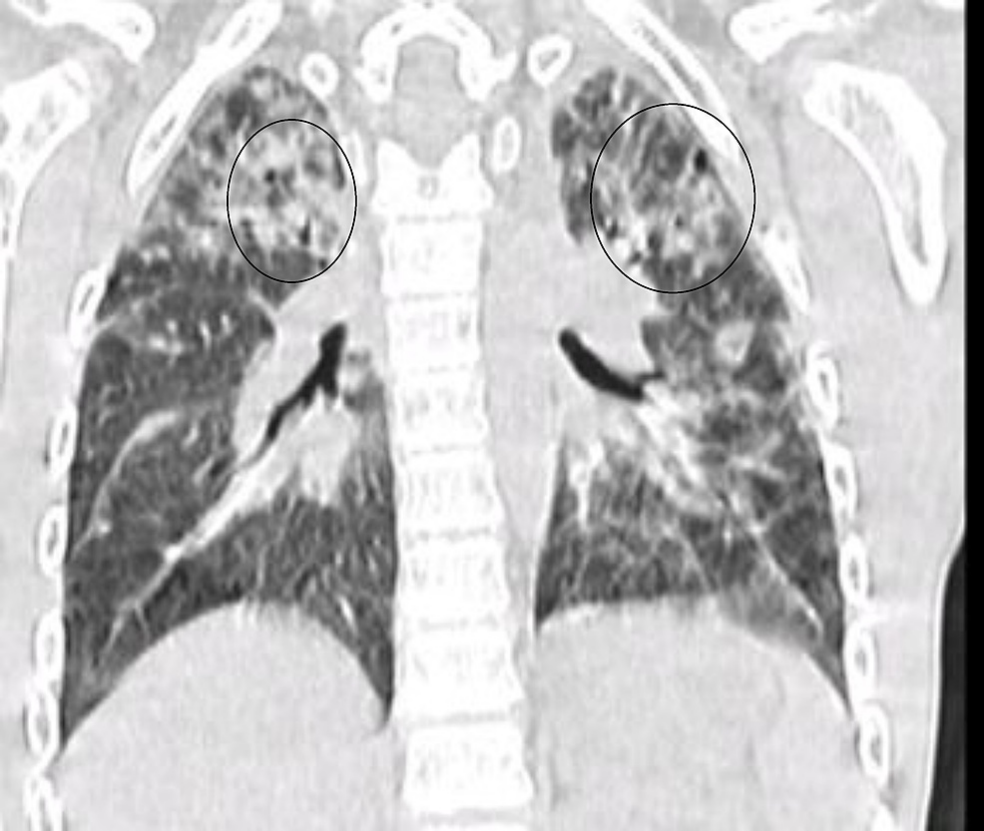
Coronal cut of high-resolution CT in 2011. High-resolution chest imaging showed a picture consistent with findings of interstitial lung disease.
